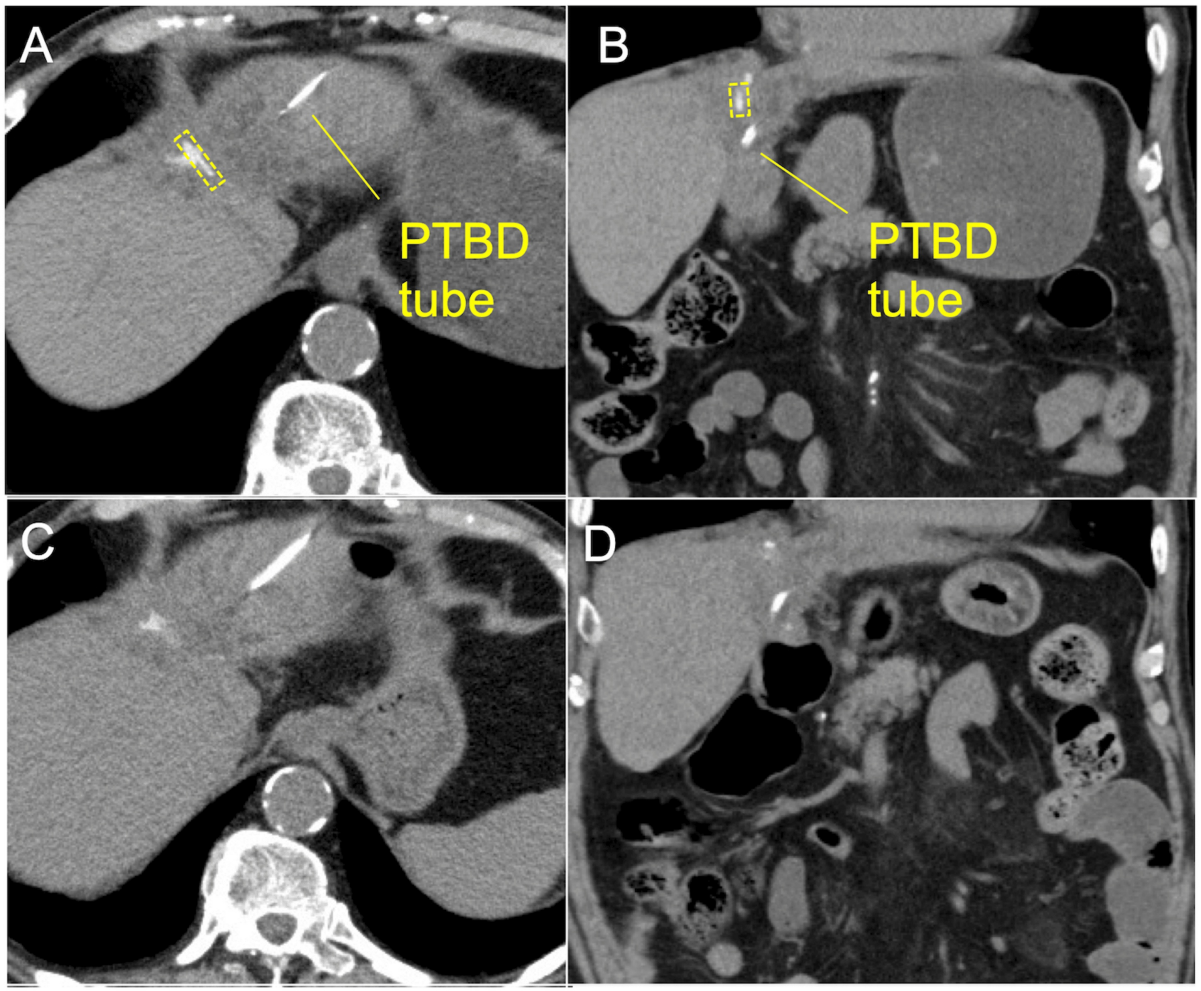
CT scans three months before and upon admission. A, B. A CT scan performed three months before admission at the level of the liver dissection plane shows a clip on the liver dissection plane (indicated by a dotted line). C, D. The clip is no longer visible at the same level on the CT scan obtained at admission. The left and right sides show the axial and coronal images, respectively. CT, computed tomography; PTBD, percutaneous transhepatic biliary drainage
